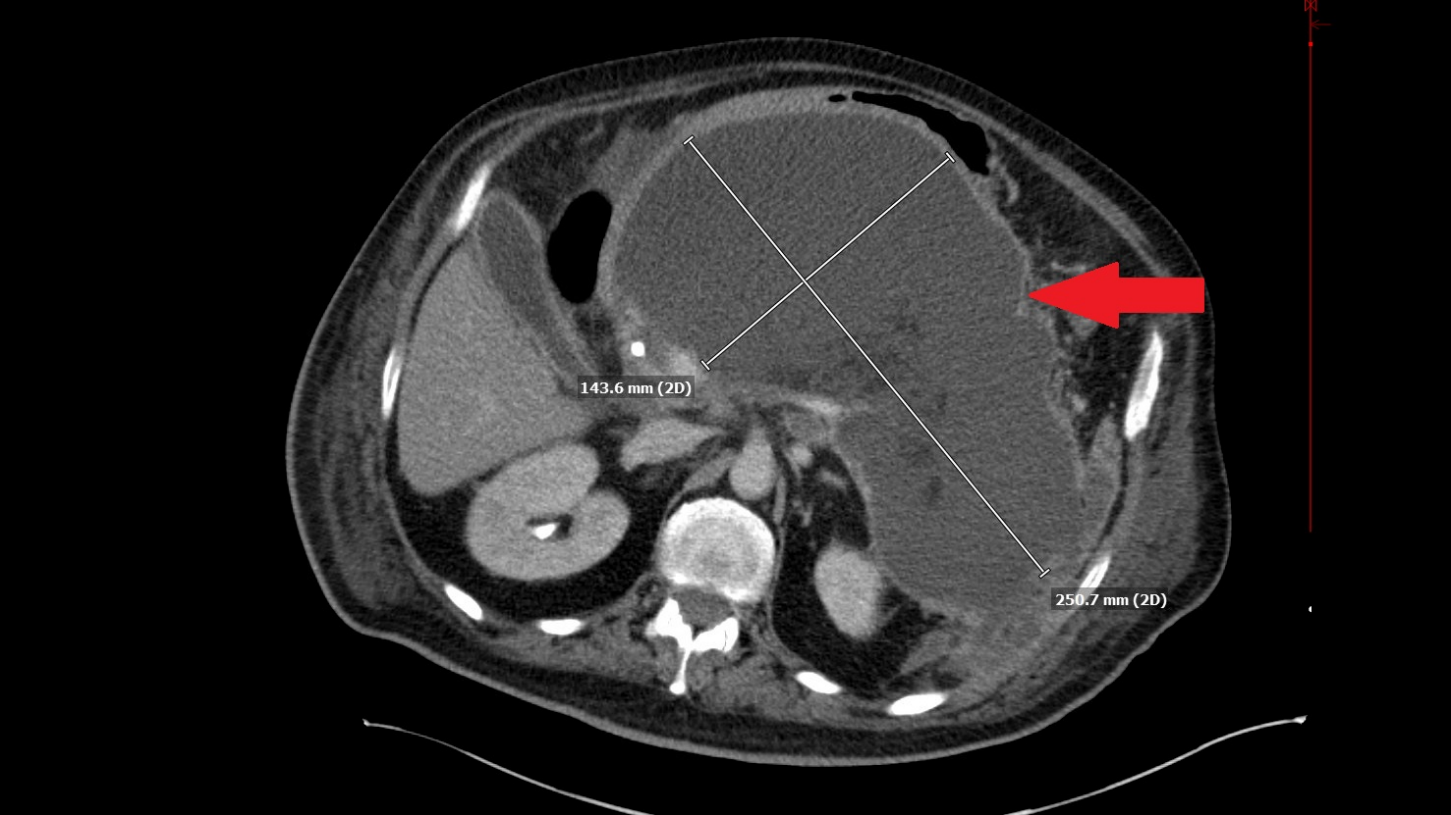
Computed tomography of the abdomen - transverse view Computed tomography of the abdomen showing a transverse view of a large pancreatic pseudocyst
